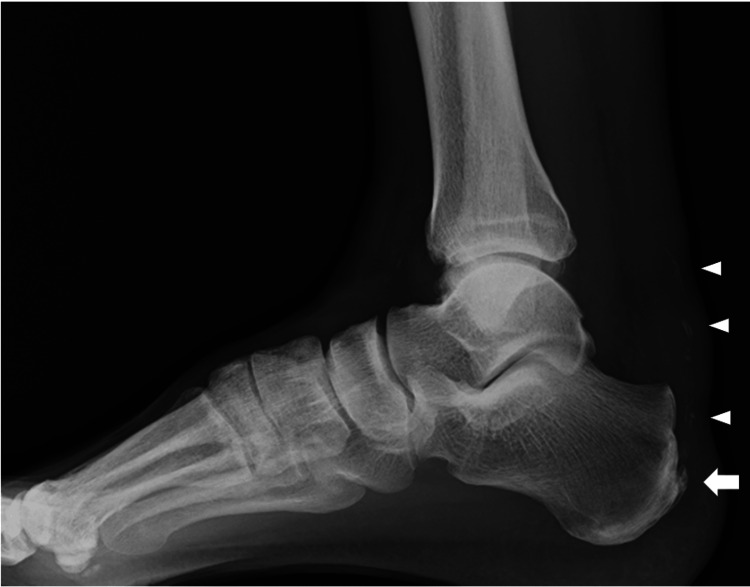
Preoperative plain radiograph A bone spur was present at the calcaneal insertion of the Achilles tendon (arrow), and a calcified lesion was identified along the course of the tendon (arrowheads).
